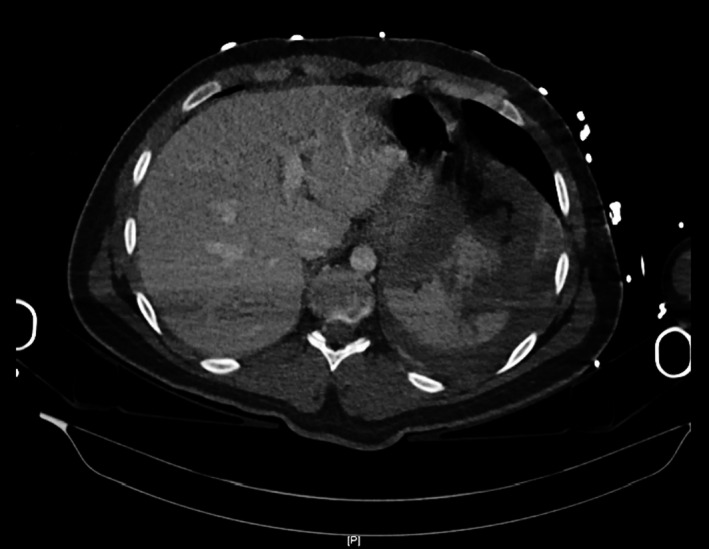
Computed tomography (CT) abdomen done at presentation. No liver abnormality seen on this scan as exemplified by this image
